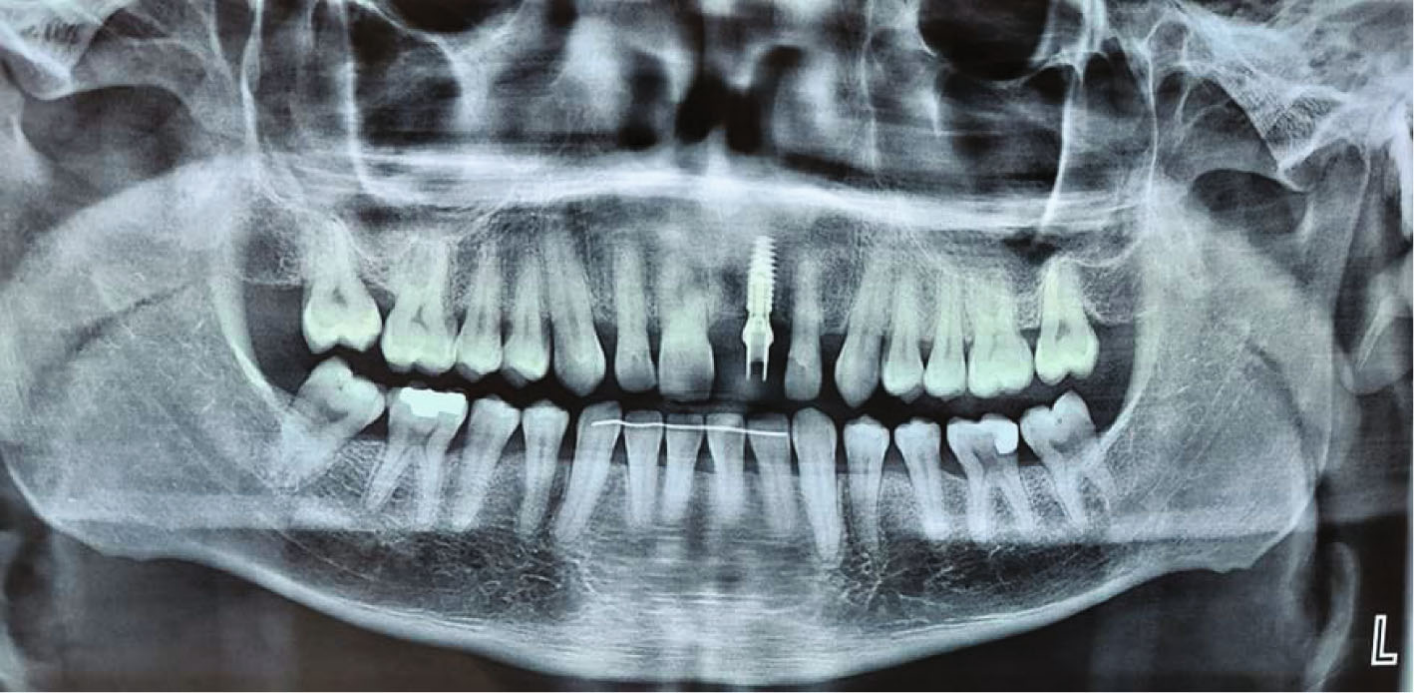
Panoramic radiographs: osseointegration of the implant at Site #21.
